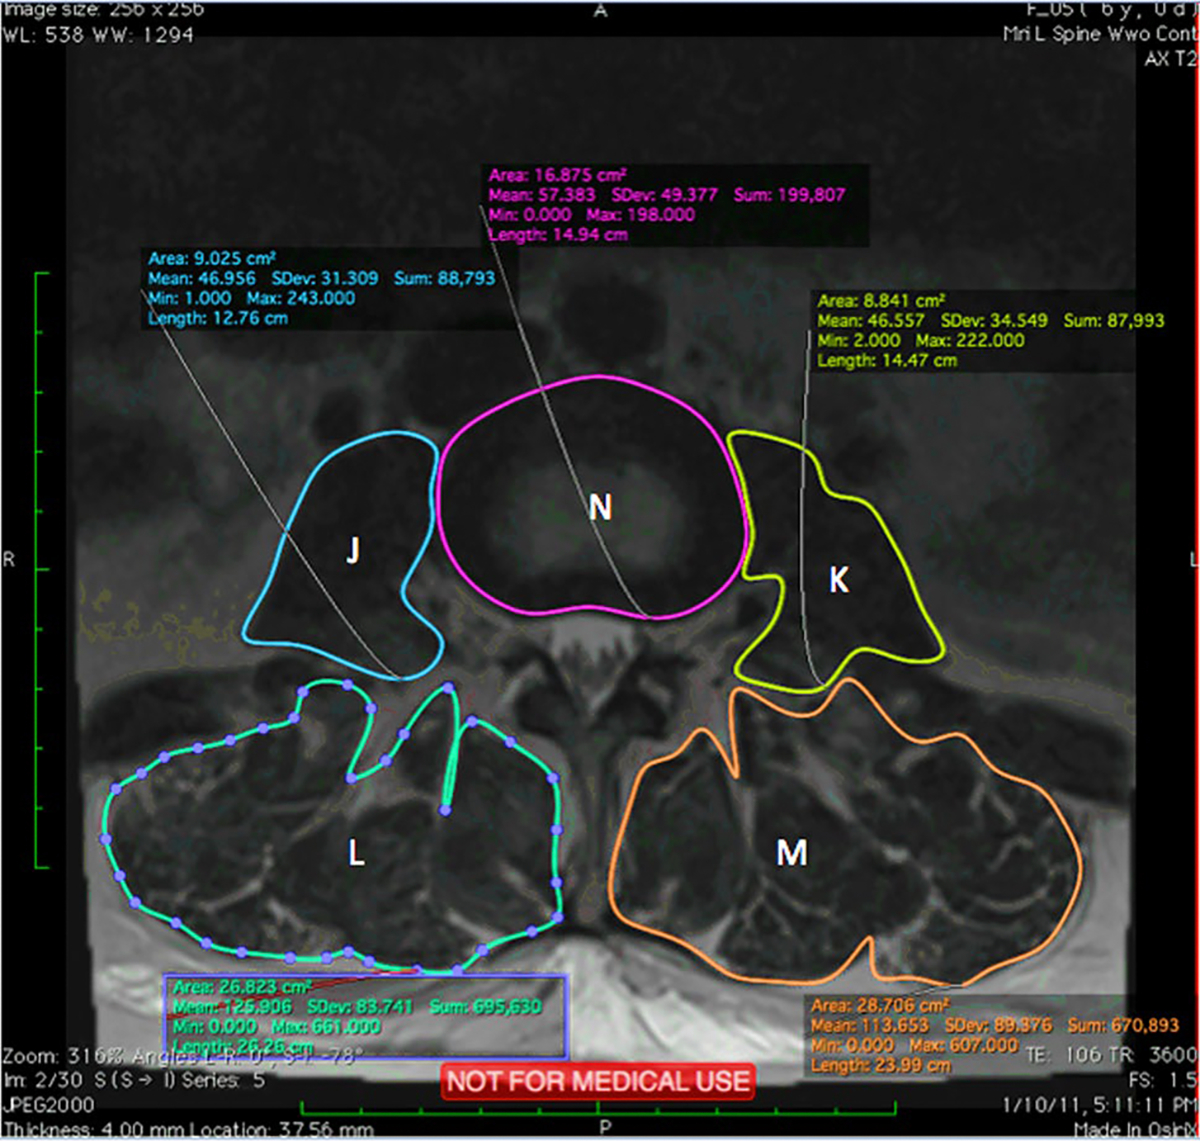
Axial MRI scan measurements of J–N
